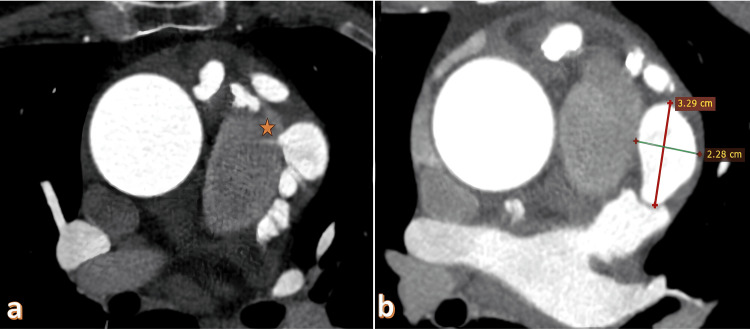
Coronary-pulmonary artery fistula and giant aneurysm. a) Axial coronary CTA shows a fistulous jet flow between the pulmonary artery and aneurysm (asterisk). b) A giant aneurysm measuring 32x22 mm is observed adjacent to the coronary-pulmonary artery fistula. CTA: Computed tomography angiography
